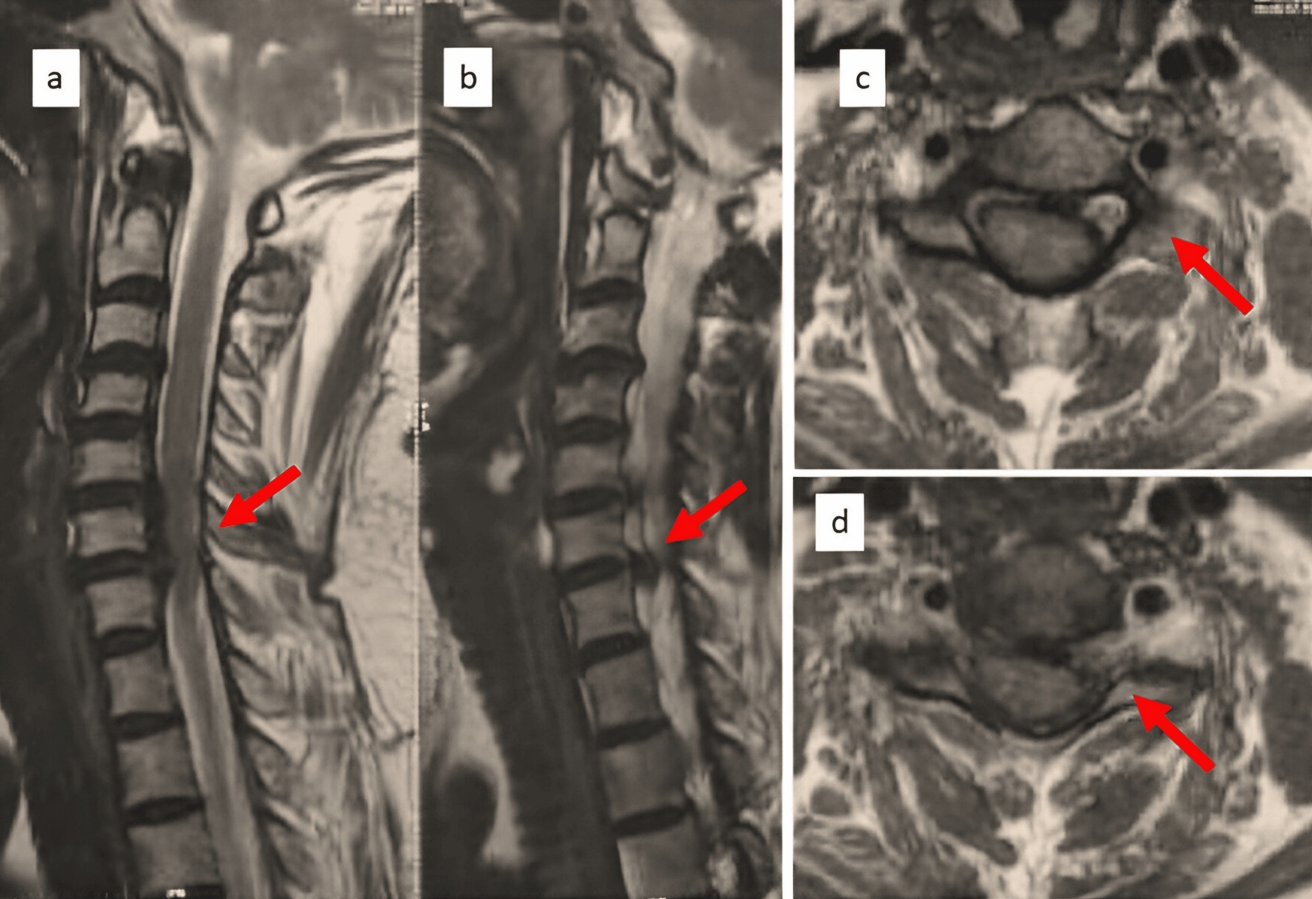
Preoperative MRI showing C5-C6 and C6-C7 prolapsed intervertebral disc. Preoperative MRI images of the cervical spine: (a-b) sagittal cut with the red arrow highlighting disc pathology; (c-d) axial MRI cut with the red arrow highlighting disc pathology.
